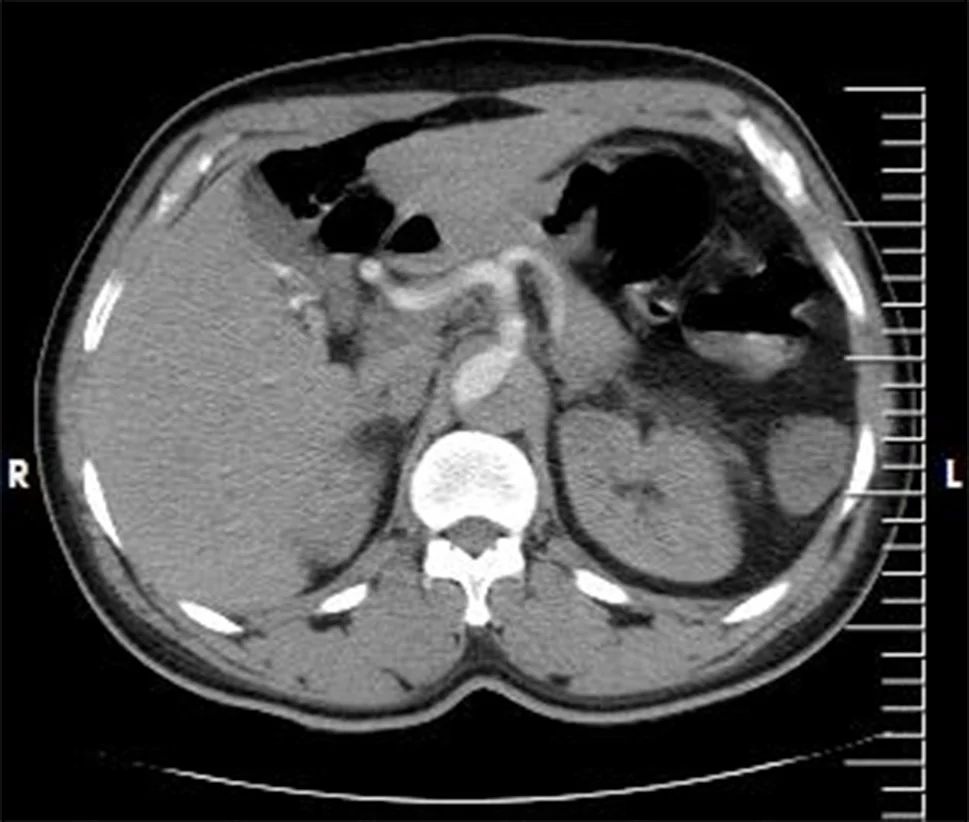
Reconstructed lower slice CT showing thrombi at the origin of the splanchnic (mesenteric) vessels which suggest the mechanism for mesenteric hypoperfusion, which clinically manifested as symptoms of bowel obstruction
